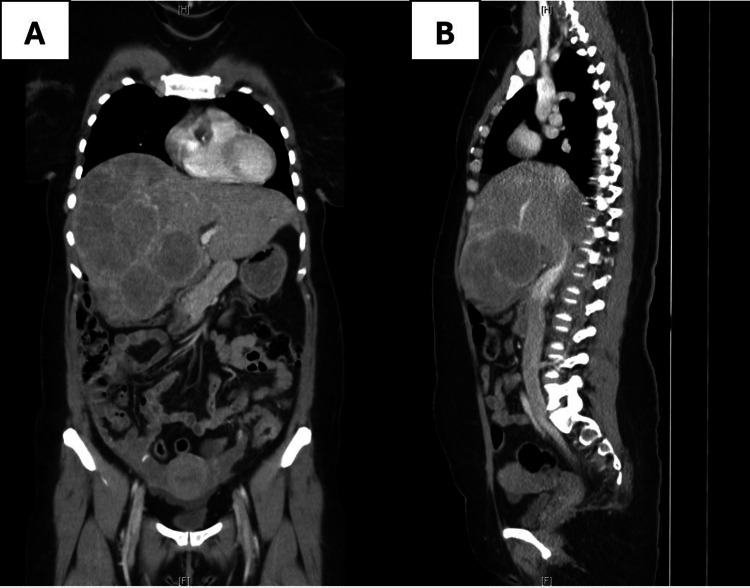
CT scan images Contrast-enhanced abdominopelvic CT scan demonstrating multiple solid hepatic nodules, highly suggestive of metastatic disease. A - coronal view. B - sagital view.
